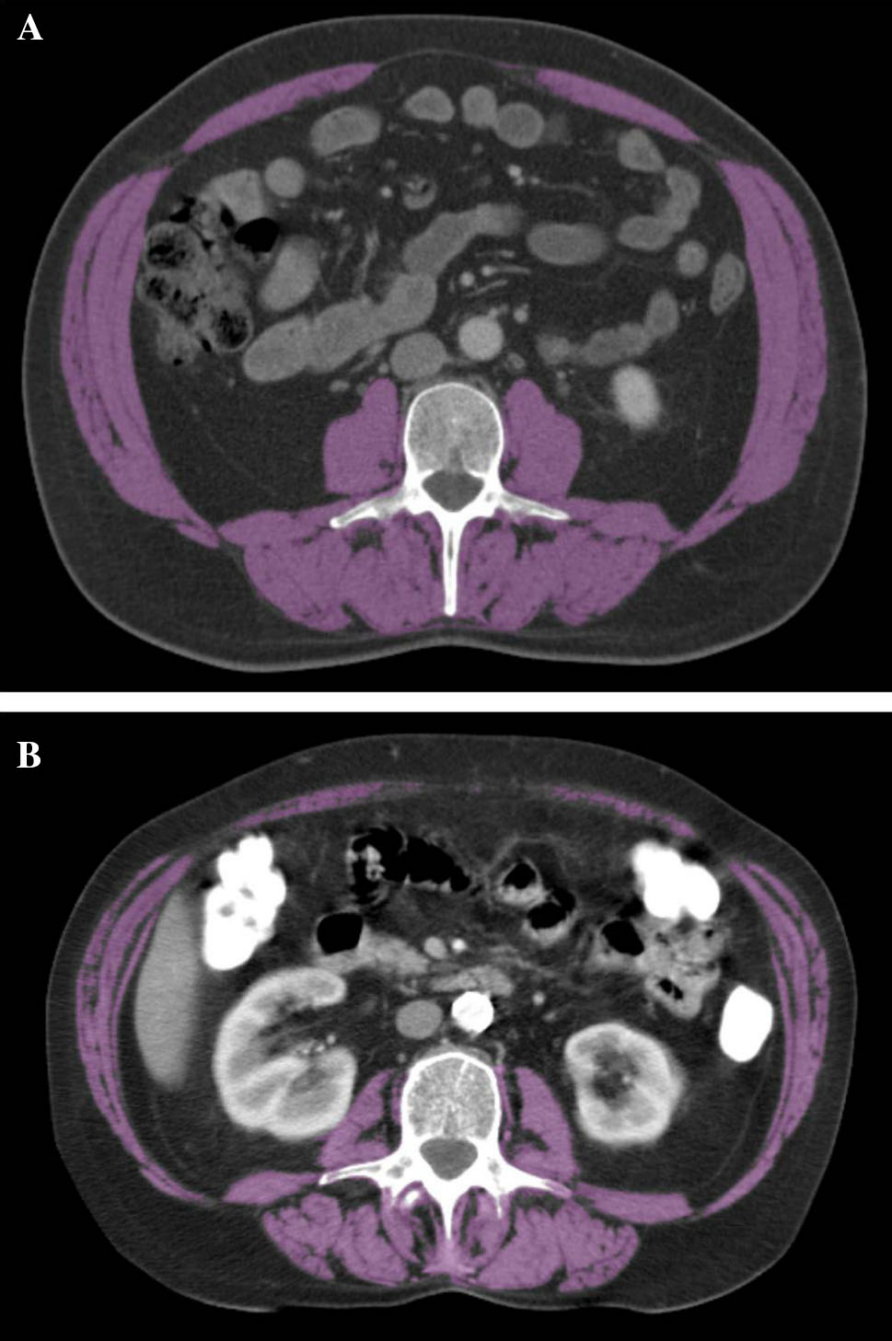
a, b. The total cross-sectional transverse area of skeletal muscles was assessed at the caudal end of the third lumbar vertebra (*highlighted in purple*) on a CT scan of both a non-sarcopenic (a) and a sarcopenic patient (b)
